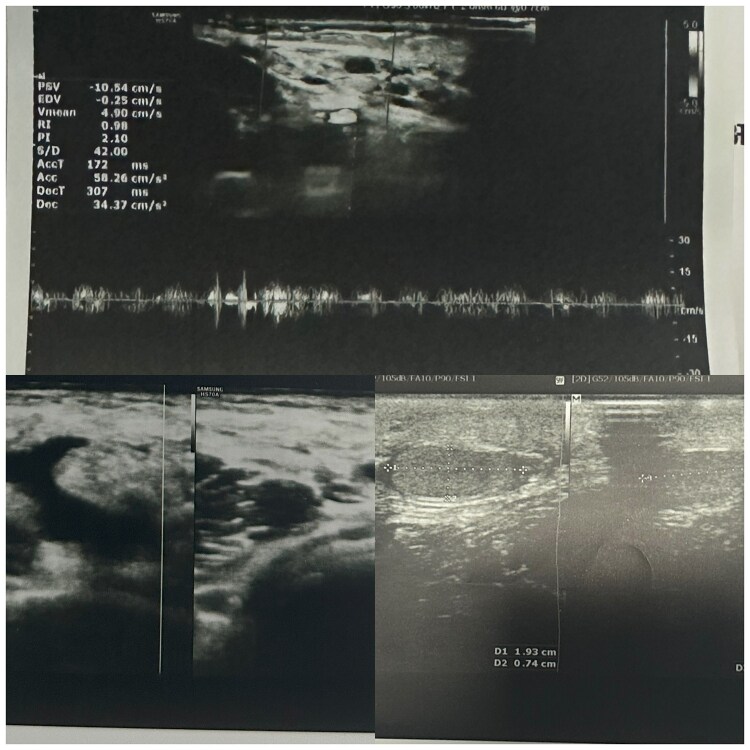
Scrotal ultrasound: heterogeneous subcutaneous lesions in the left thigh, lower abdominal wall and scrotum, with multiple cystic spaces showing venous flow.
